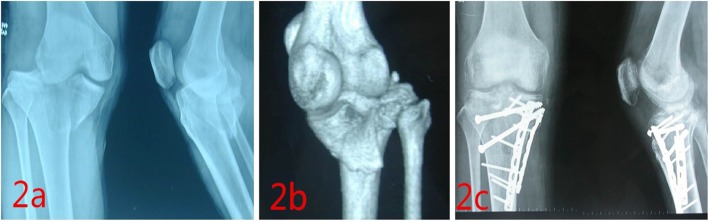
The figure showed a 35-year-old man (case 1) who had the Schatzker type IV right tibial plateau fracture involving posteromedial plane. a The radiograph of the patient before the operation. b The three-dimension reconstruction image of the patient before the operation, and it showed the Schatzker type IV tibial plateau fracture involved posteromedial plane. c The fracture was fixed by dual plates, with one plate fixing the medial fragment and one fixing the posterior fragment. The avulsion fracture of ACL was fixed by lag screws
